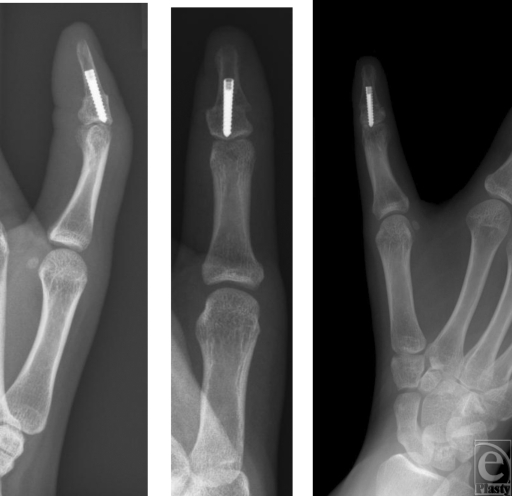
Postoperative radiographs at 12 weeks.
